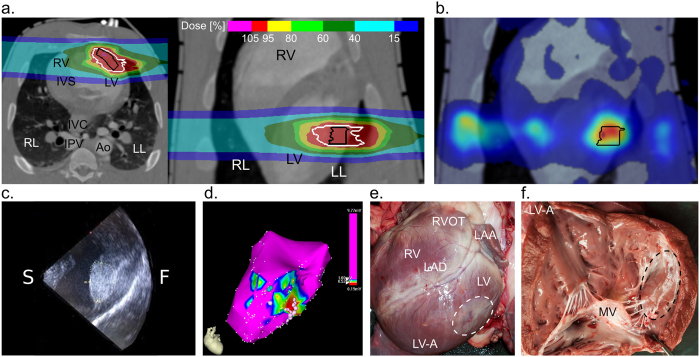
Outcomes after Left Ventricular (LV) Freewall Irradiation. (a) Transverse and coronal plane of a treatment plan with two lateral carbon beams (40 Gy). Details as described for Fig. 1a. (b) Anteroposterior view of by-product β^+^-activity during irradiation *via* PET, superimposed on the coronal plane of a contrast-enhanced CT scan. Black contour = target contour, β^+^-activity shown as color-wash. (c) Intracardiac ultrasound image of the LV freewall after irradiation, hyperechoic lesion area marked by dotted lines. (d) Left anterior oblique view of an endocardial voltage map of the LV 6 months after irradiation. As indicated in the legend, local voltage >1.0 mV is depicted in magenta. Voltage <0.5 mV in red. Other colors mark voltages in-between. White dots = fragmented potentials. (e) Macroscopic LV epicardial lesion outcome six months after irradiation with the dashed line marking the contoured target zone. (f) Endocardial lesion outcome six months after irradiation. Ao = Descending aorta; F = Freewall; LAA = Left atrial appendage; LAD = Left anterior descending coronary artery; LIPV = Left inferior pulmonary vein; LVOT = Left ventricular outflow tract behind the mitral valve leaflet; LL = Left lung; IVC = Inferior vena cava; LV = Left ventricle; LV-A = Left ventricular apex; RIPV = Right inferior pulmonary vein; RL = Right lung; RV = Right ventricle; S = Septal site.
